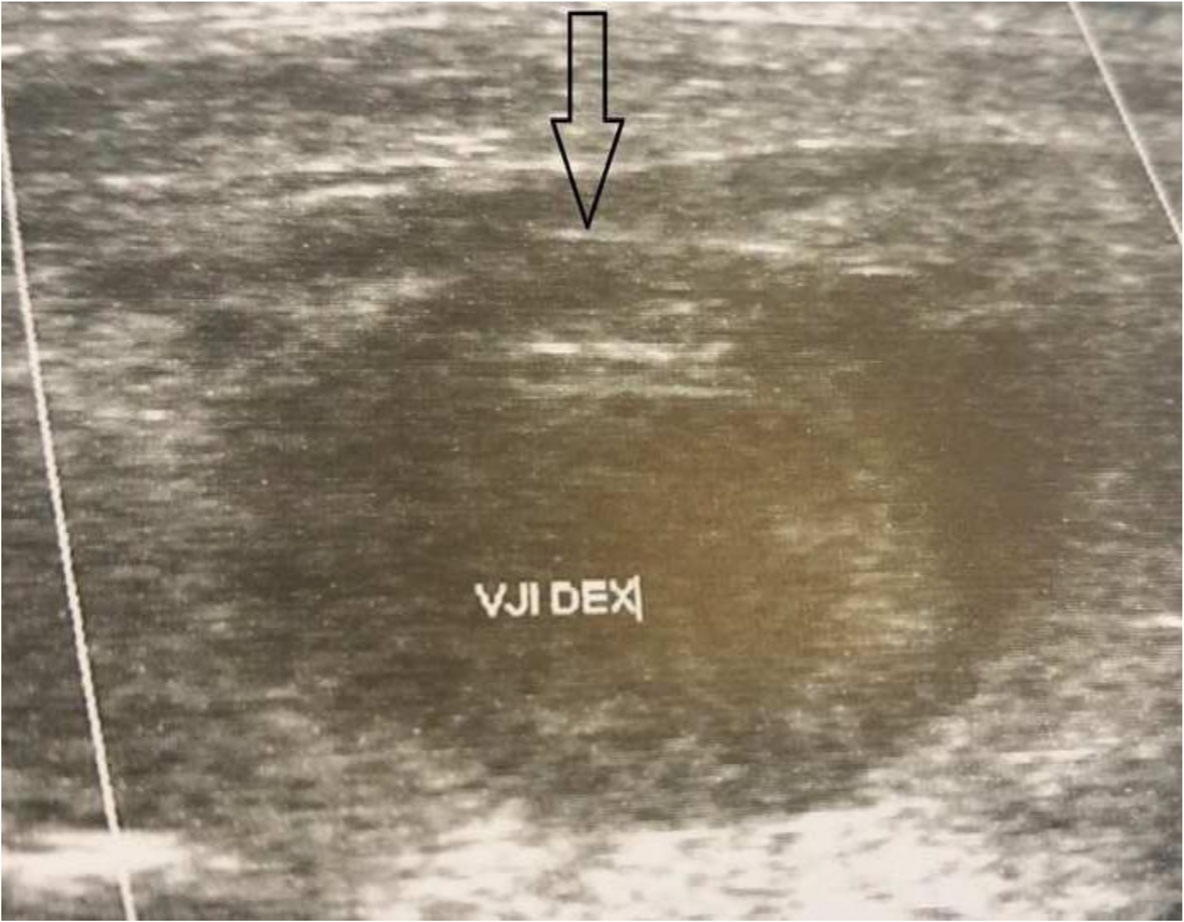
Doppler ultrasound of the right internal jugular vein (arrow) showing filling defect suggestive of thrombosis
